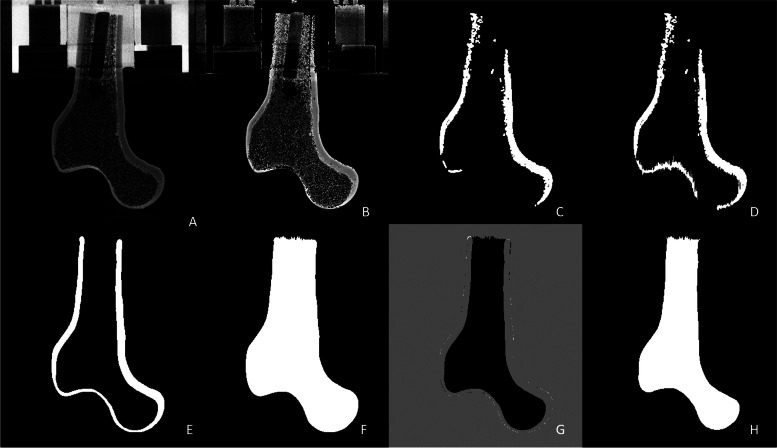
Steps of Segmentation in terms of Midplanes of SYN. A rescaled image showing the truncated bone with its embedding and the sample holder used for the μ-CT scanning B usage of a global threshold to get rid of the embedding as well as the sample holder C usage of a morphological filter to separate the cortex and the spongiosa D first manual segmentation in 3D Slicer E smoothed final manual segmentation of the cortex F whole bone mask G subtracted cortex from the whole bone to get the spongiosa H grey value inverted spongiosa
